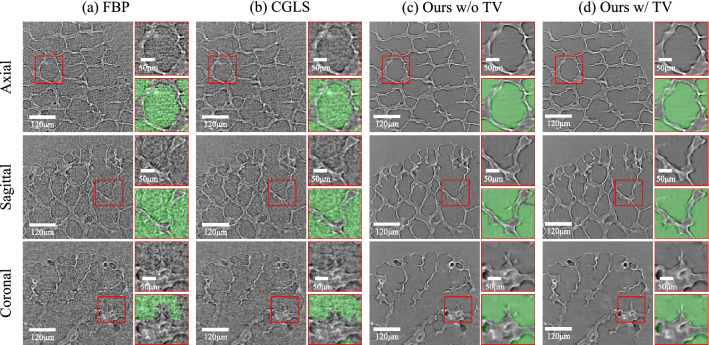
Qualitative results of synchrotron X-ray projection dataset using (a) FBP^26^, (b) CGLS^31^, (c,d) our framework without or with TV term. Red boxes indicate specific ROI of reconstructed images and segmented images.
